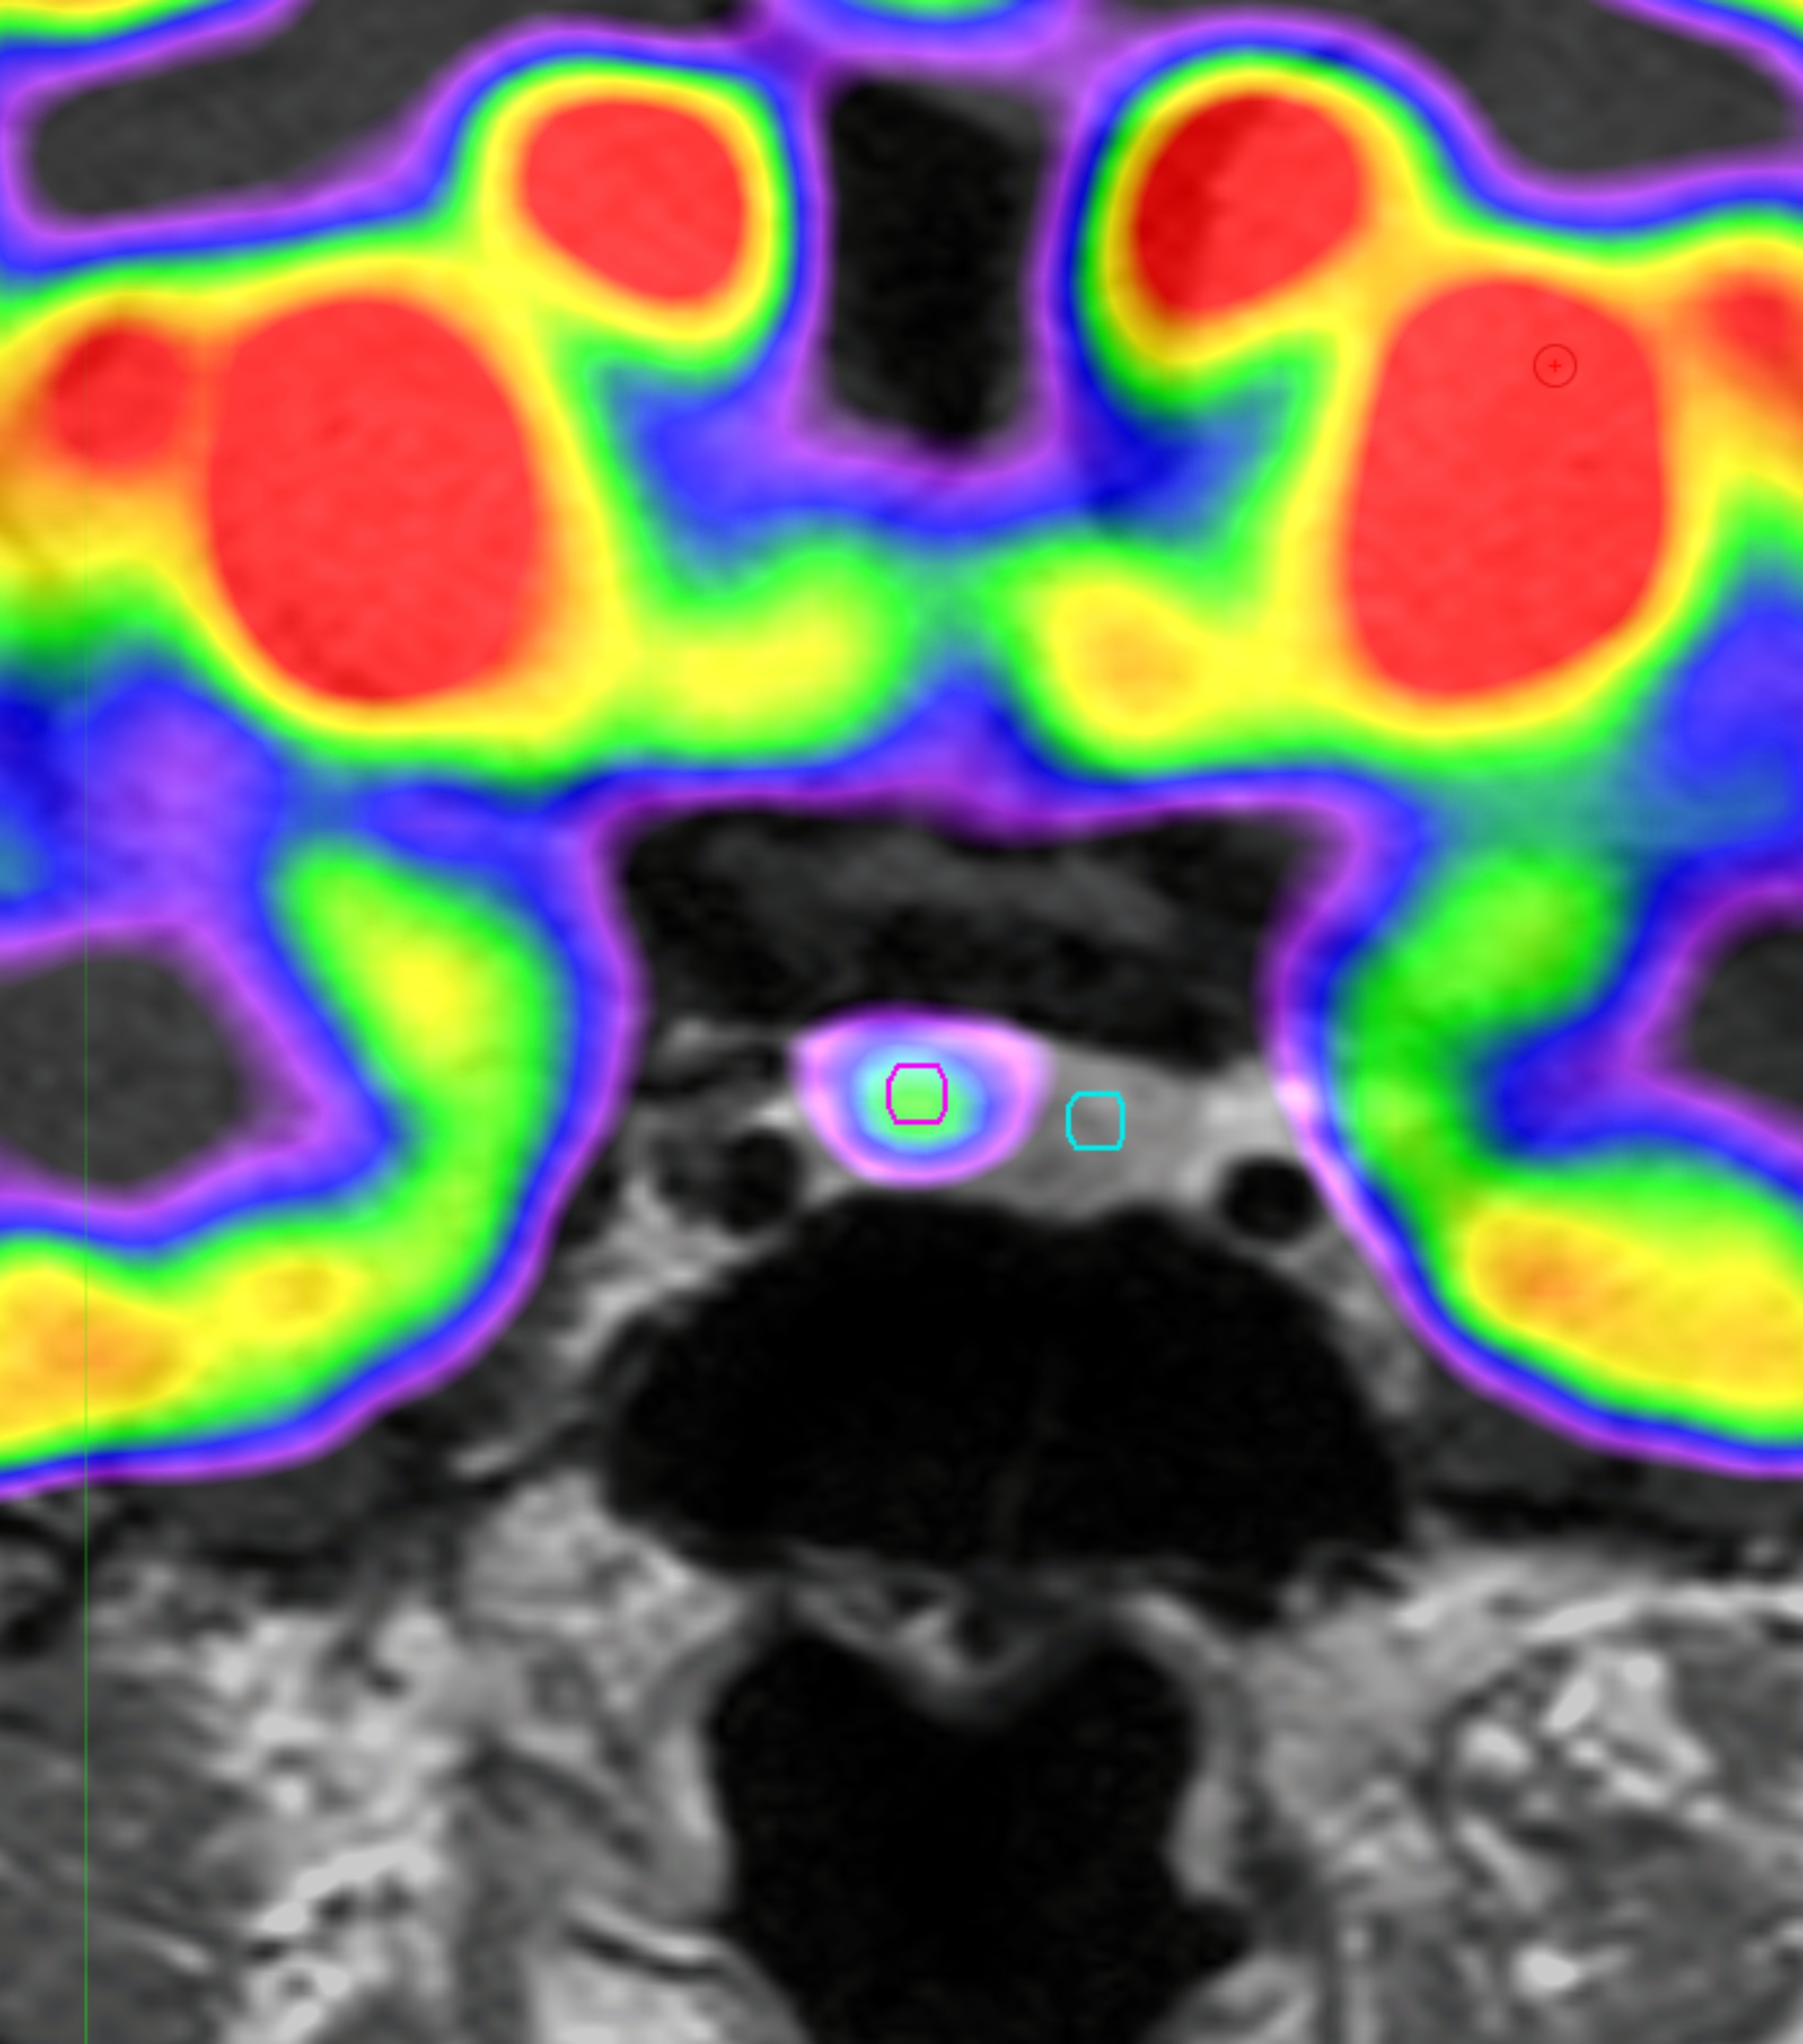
Images of ROI for pituitary adenoma on^18^F-FDG PET scan Example of ROI definition in pituitary adenomas of ^18^F-FDG PET scan of the patients with CD. We draw the fixed circular ROI with a 3-mm diameter for pituitary adenomas (red circle) and normal pituitary gland (green circle) ROI, Reason of interest; ^18^F-FDG PET, ^18^ F-fluorodeoxyglucose positron emission tomography; CD, Cushing disease
